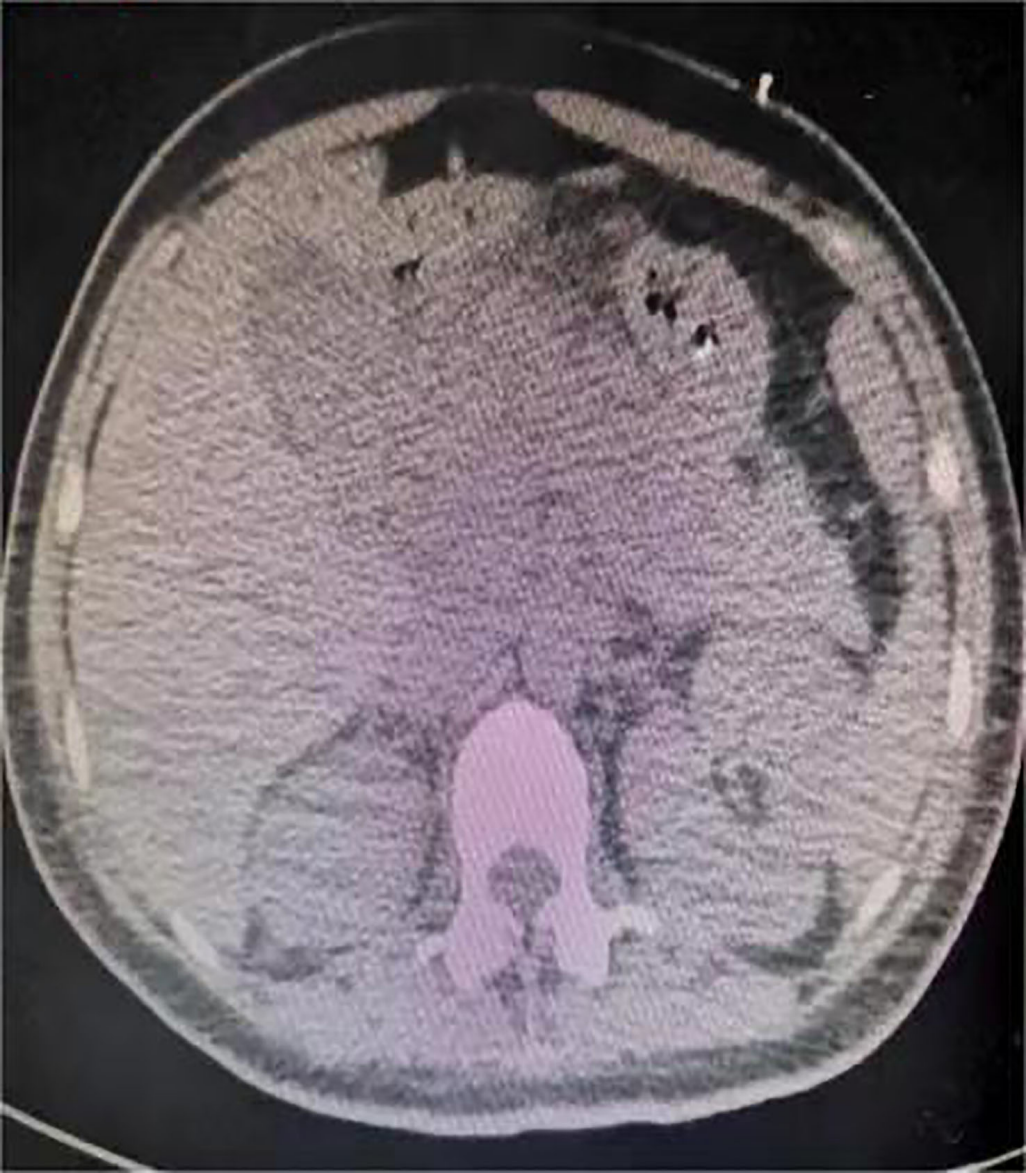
Computed tomography of the patient's abdomen after cesarean section.
